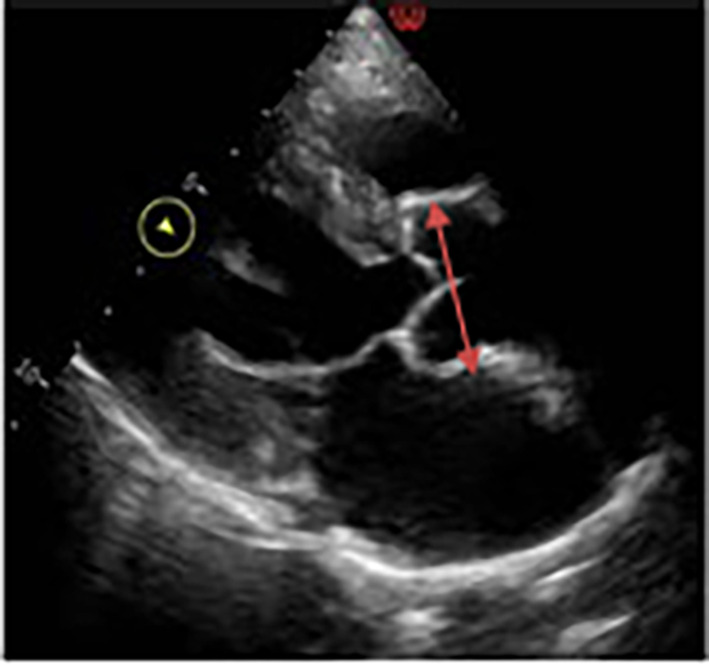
2D echocardiogram showing non‐involvement of the aortic root and part of the ascending aorta.
